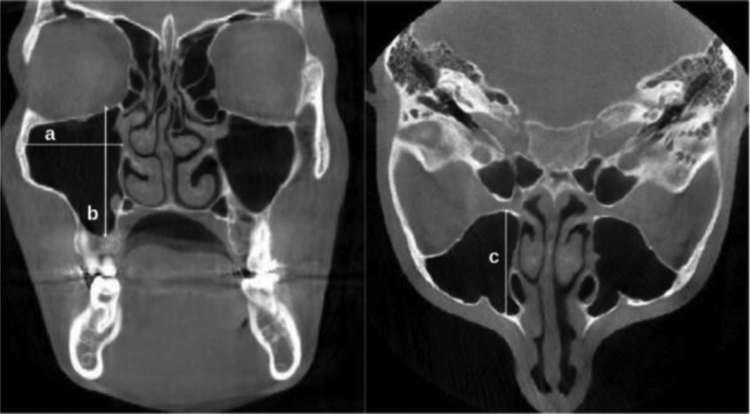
The coronal and axial CBCT images revealing the measurement of the MSW, MSH, and MSL. MSW; the longest distance from the most medial wall of the sinus to the most lateral wall of the sinus (a), MSH; the longest distance between the sinus floor and the sinus roof (b), MSL; the longest distance between the anterior wall and the posterior wall of the sinus (c).
